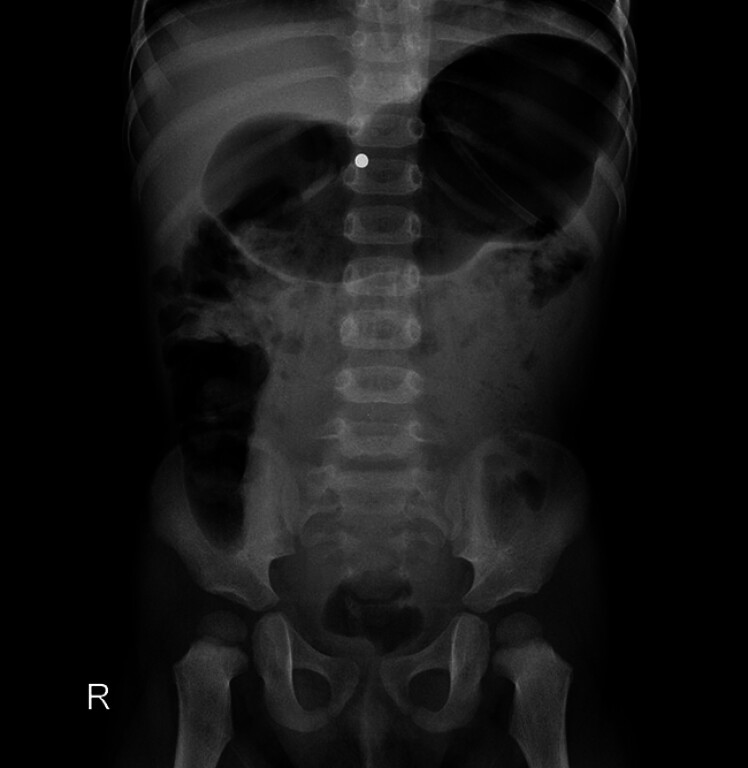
Abdominal X-ray showing a magnetic bead inside the body.
